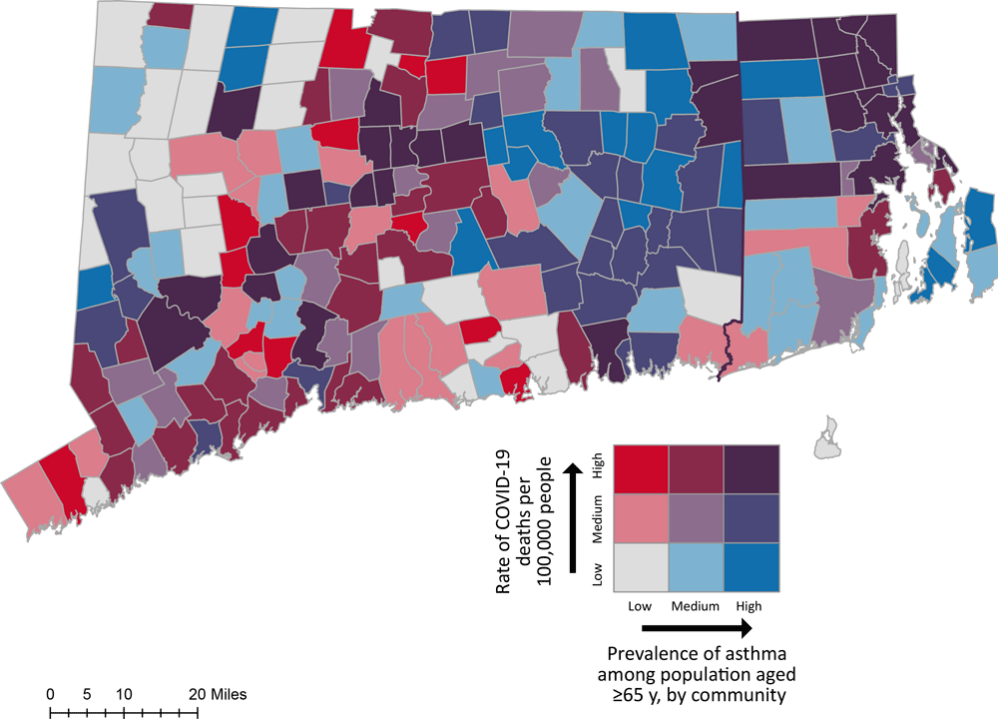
Town-level prevalence of asthma among population aged 65 years or older and COVID-19 death rates per 100,000 people, Connecticut and Rhode Island. Data sources: Connecticut Department of Public Health (8), Rhode Island Department of Public Health (9), HealthyAgingDataReports.org (10,11), CT DEEP GIS (22), and RIGIS (23).
